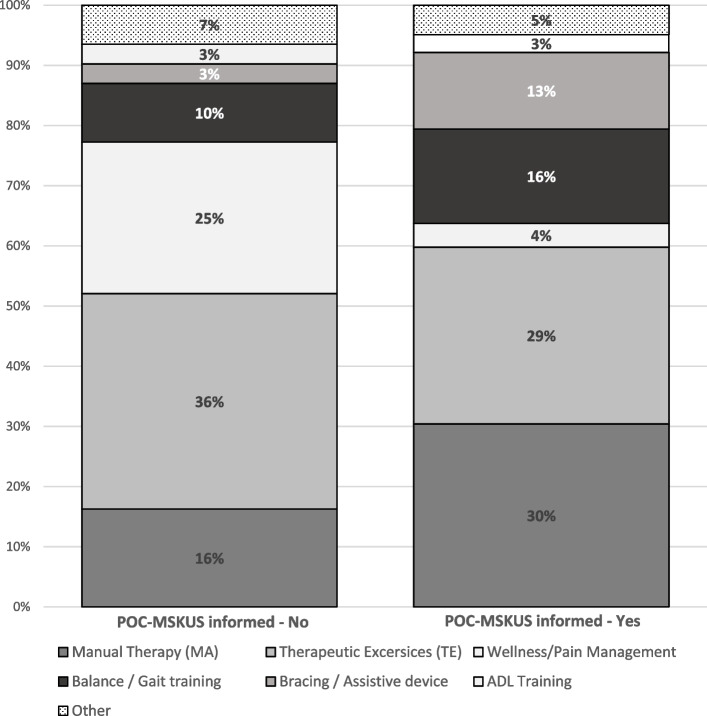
Proportion of physical therapy items in plans informed (*n* = 102) and not informed (*n* = 123) by Point-of-Care Musculoskeletal Ultrasound (POC-MSKUS).
